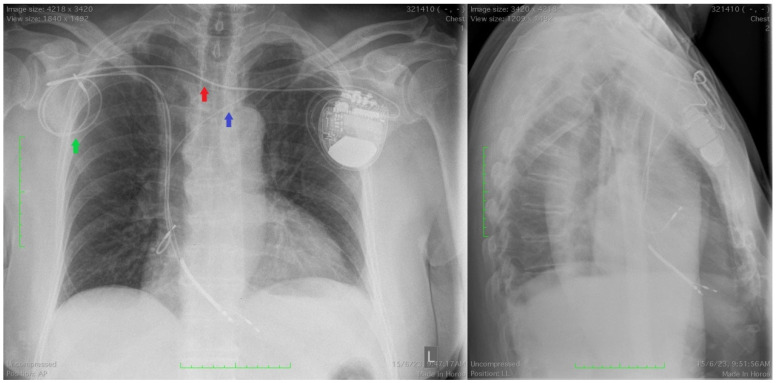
Legend: Post-procedural chest X-ray 24 h after implantation showing the tunneled presternal atrial lead (red arrow), the abandoned right-sided ventricular lead (green arrow) and the left--sided defibrillator lead (blue arrow).
